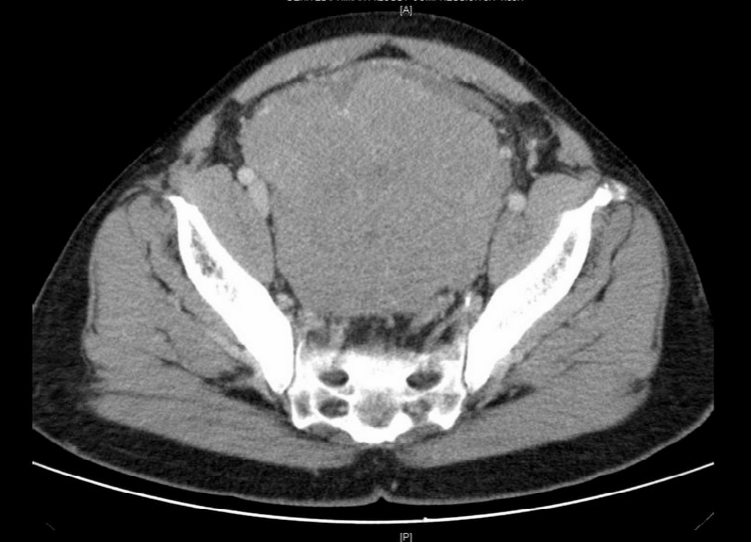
CT scan demonstrating a 12 × 13 × 10 cm (1560 cm3) mass in the pelvis. There is significant bilateral compression of the external iliac vessels and rectum.
